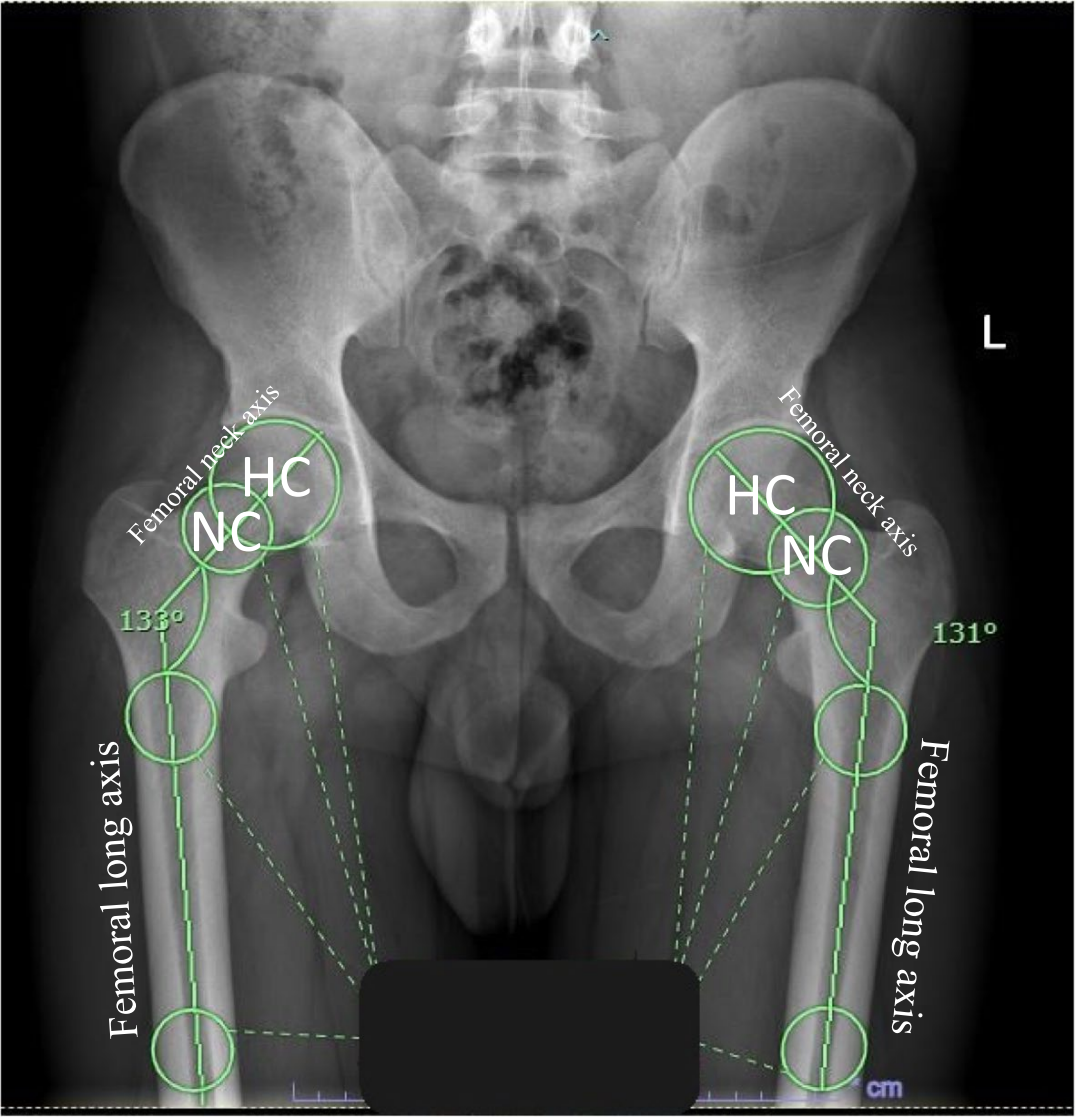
The measurement method of neck-shaft angle on upright pelvis plain radiograph. HC, femoral head center; NC, femoral neck center
